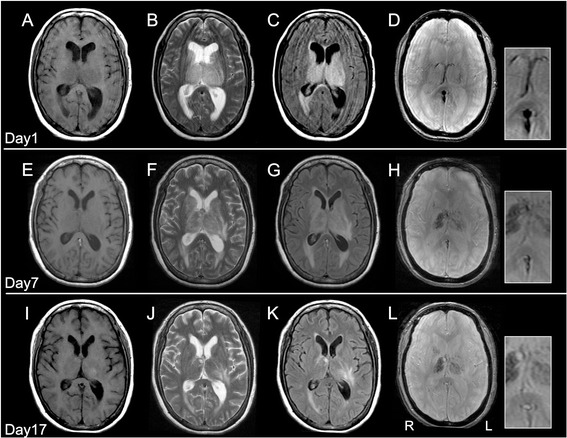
Brain magnetic resonance images. Magnetic resonance imaging was performed at admission (a–d), and 7 days (e–h), and 17 days (i–l) after admission. At admission, the thalamus and caudate nuclei were involved bilaterally with edematous swelling, demonstrated on magnetic resonance imaging by a hypointensity of T1-weighted signal, b hyperintensity of T2-weighted signal, and c hyperintensity of fluid-attenuated inversion recovery signal. d Diminished signal and enlargement of the deep cerebral veins and the straight sinus are demonstrated in a T2*-weighted image. e–g At day 7 after admission, small hyperintense areas were observed in the anterior and left posterior regions of the thalamus in a T1-weighted image (e), and a worsening of brain swelling was demonstrated in T2-weighted (f) and fluid-attenuated inversion recovery signal (g) images. h A T2*-weighted image showed hemorrhagic changes in the thalamus with right-side predominance, but abnormal signal of deep cerebral veins was less prominent than at admission. i–k At day 17, although the size of the T1-hyperintensity area in the left thalamus had increased from day 7 (i), the edematous swelling improved (j, k). l Abnormal signal of deep cerebral veins was not present in a T2*-weighted image
